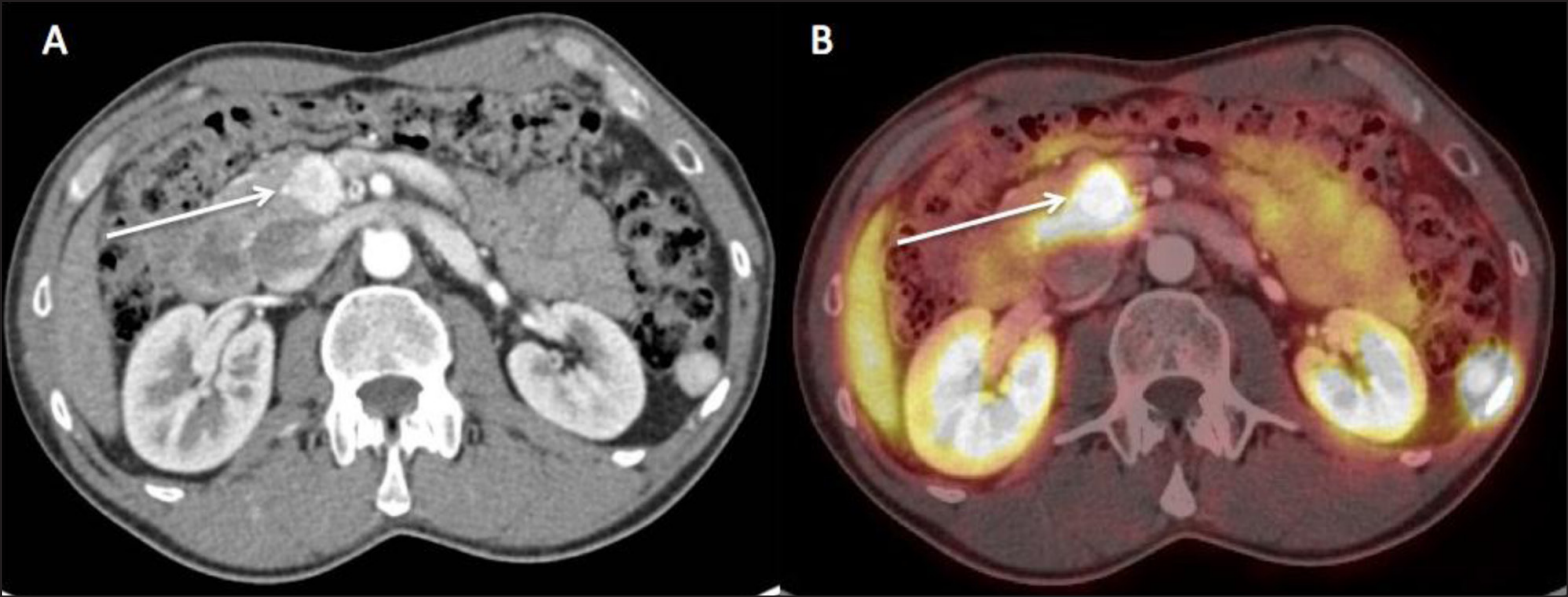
PET images showed a focus of uptake in the pancreas (Figures 1B and 2A, arrows) and moderate tracer uptake foci (arrows) in the spinous process of the first thoracic vertebra (Figures 2B and 3A), the bodies of the fifth and eighth thoracic vertebras (Figures 2C, 3B, and 3C), and the right iliac wing (Figures 2D and 3D). These uptakes of ^68^Ga DOTATATE could be taken for bone metastases. The corresponding CT images, however, showed characteristic appearances of hemangiomas, consisting in bone demineralization with vertical striation due to thickened trabeculae (“Corduroy sign” on the sagittal planes) and a “polka-dot” appearance on the axial slices where the thickened trabeculae are seen as small punctate areas of sclerosis (Figure 3, arrows).
